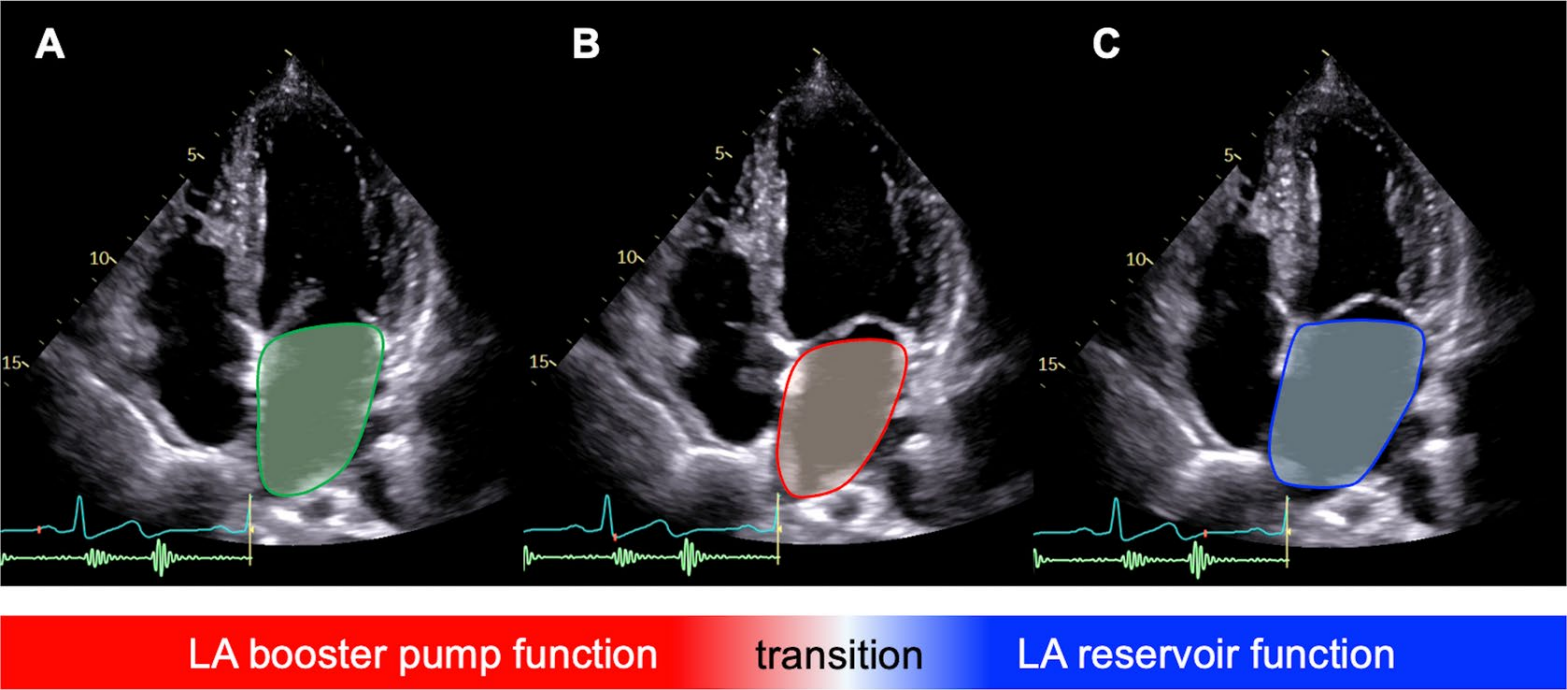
LA volumetric change from the apical four chamber view. Figure shows LA volumetric change from the apical four-chamber view. At the onset of P-wave A, the LA myocardium starts to contract for minimizing LA volume at the QRS complex B. The volumetric change in this phase (LA active emptying fraction) is a parameter of LA booster pump function. Immediately after LA contraction, the LA myocardium relaxes due to LA active relaxation and passive dilatation to reach the LA maximal volume C. The volumetric change in this phase (LA total emptying fraction) is a parameter of LA reservoir function. LA, left atrial
